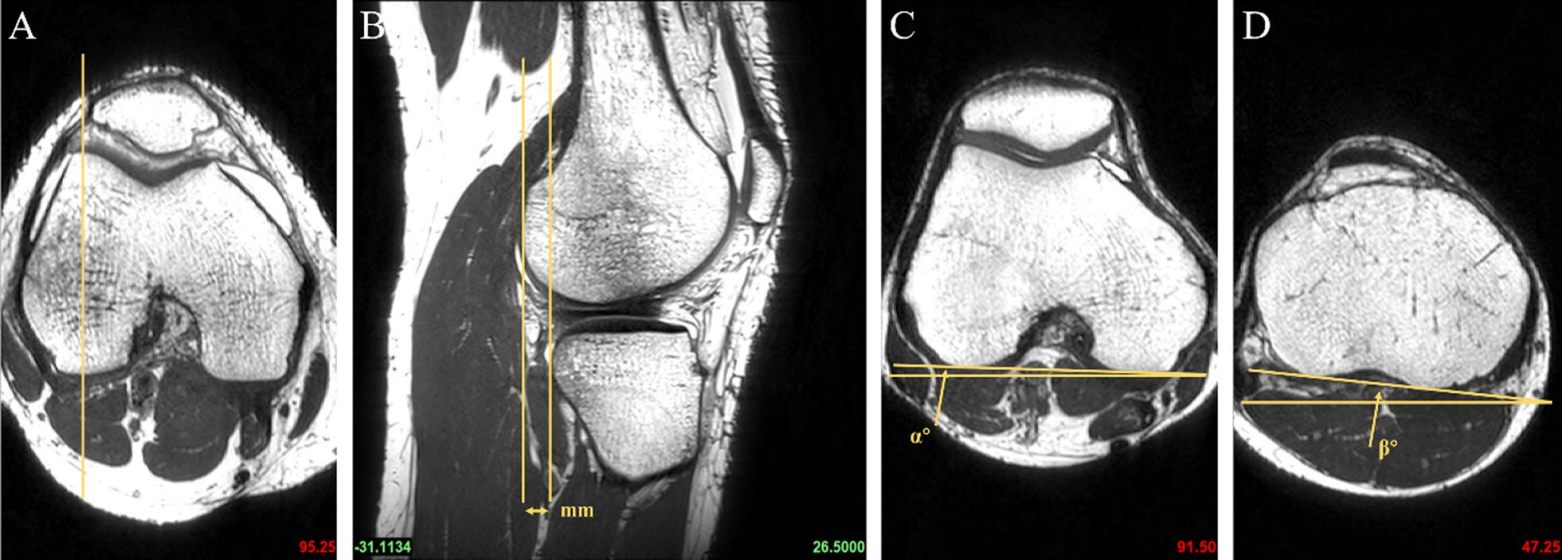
The midpoint of the lateral femoral condyle was identified on the axial MRI image (A). Two parallel lines tangent to the posterior cortex of the lateral femoral condyle and the posterior tibial cortex are made on the corresponding sagittal image (B). The horizontal distance between these two lines is the ATT (unit: millimeter) (if the tibial posterior cortex tangent line is located before the femoral posterior cortex tangent line, it is recorded as negative, indicating anterior tibial displacement; vice versa, it is positive). The widest MRI image level in the anteroposterior direction of the femoral condyles was first determined and then determine the first axial MRI image above the fibular head. The angle between the tangent line of the posterior cortex of the femoral condyle and the horizontal line was defined as the femoral angle (C). The angle between the posterior cortical tangent of the tibial condyle and the horizontal line was defined as the tibial angle (D). Internal rotation was considered positive and external rotation negative. The FTR was obtained by measuring the absolute difference between these two angles
